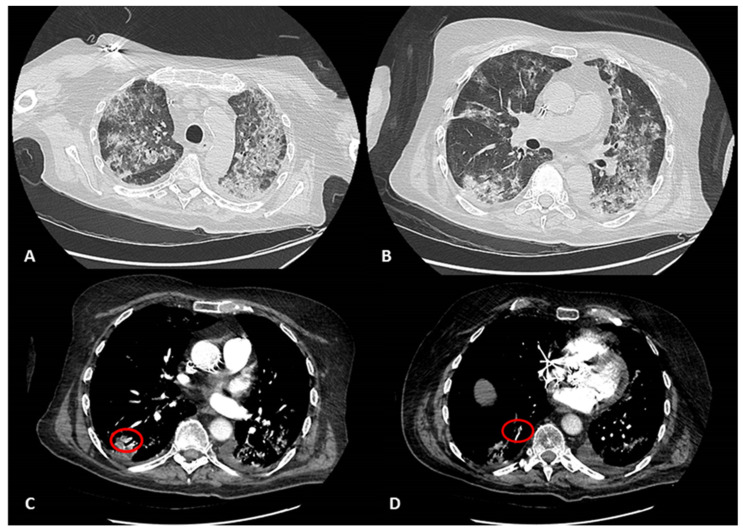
(A,B) CT scan of the chest showing multiple large bilateral patchy ground-glass opacities (GGOs) and consolidations, mostly involving peripheral lung parenchyma. (C,D) CT scan of the chest with iodinated contrast. Red circles: Thromboembolic alterations involving sub-segmentary pulmonary arteries.
